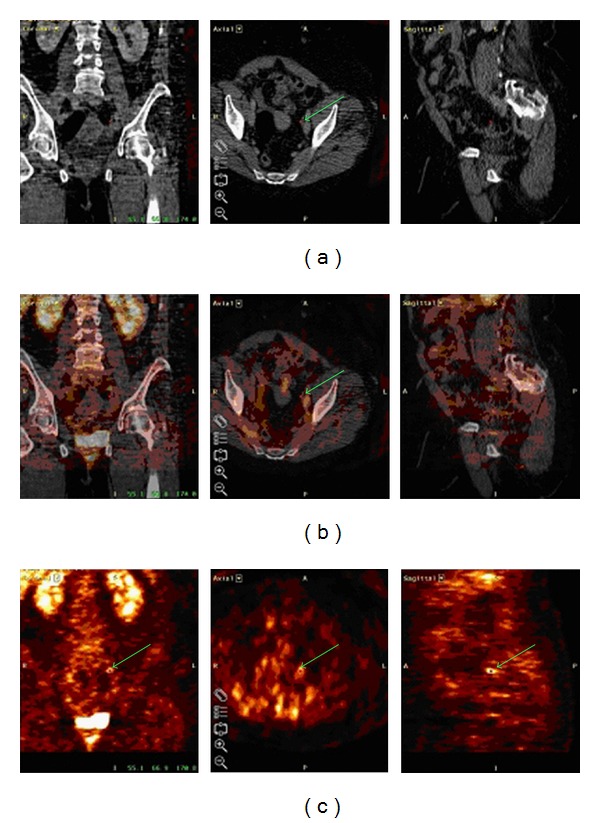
First row: computed tomography image; second row: software-based image fusion; third row: F18-FDG PET. Axial, coronal, and sagittal reformatted images of a patient with malignant lymphoma. F18-FDG PET (third row) shows a false-positive abdominal lesion (it was also scored as false positive) which turned out to be related to ureter-activity as showing by image fusion of PET with CT (second row). CT images did not show any pathologic enlarged lymph node. (first row).
